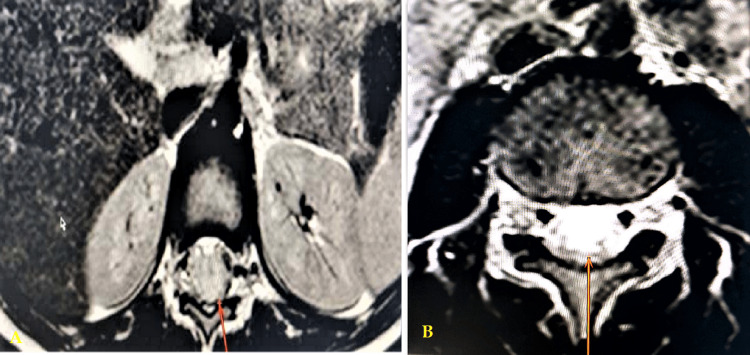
Post-contrast axial MRI T1W images Post-contrast axial MRI T1W images demonstrate the involvement of the ventral surface of the spinal cord A and B (arrows).
